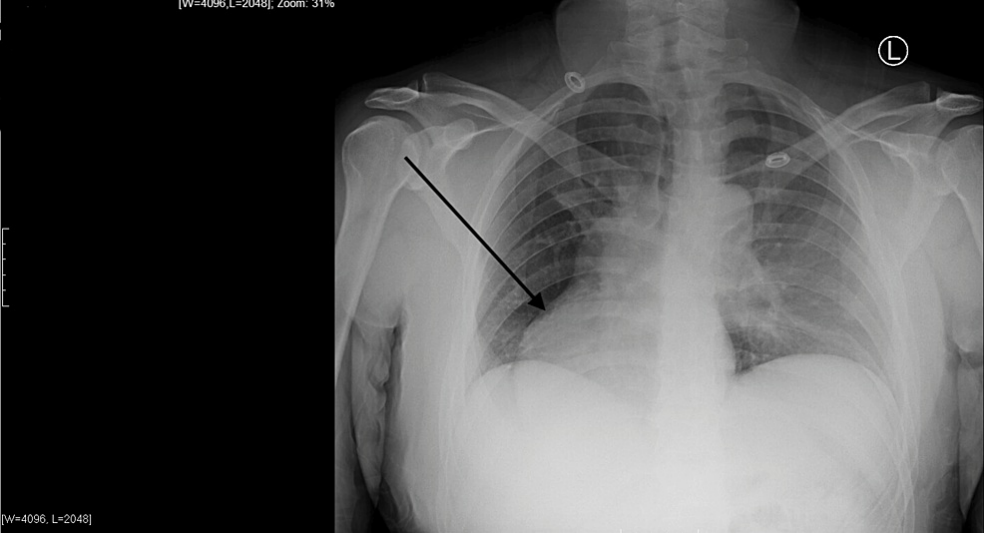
Chest X-ray demonstrating dextrocardia depicted by the arrow, degenerative changes of the bilateral shoulders, and chest wall asymmetry.
